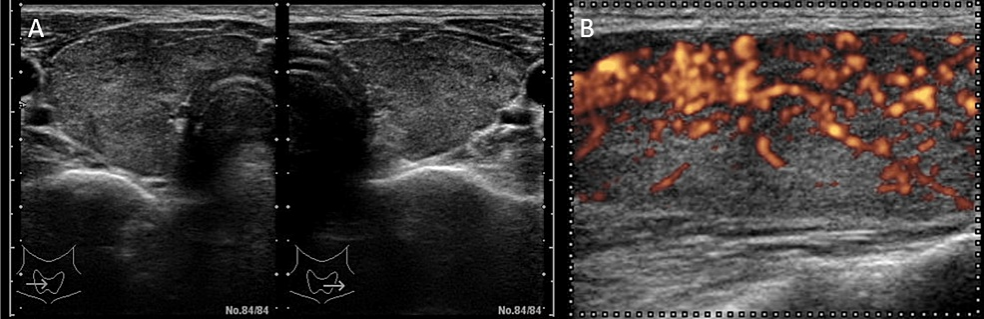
Diffuse goiter on ultrasonic examination A: Diffuse goiter. B: Thyroid gland with increased blood flow.
